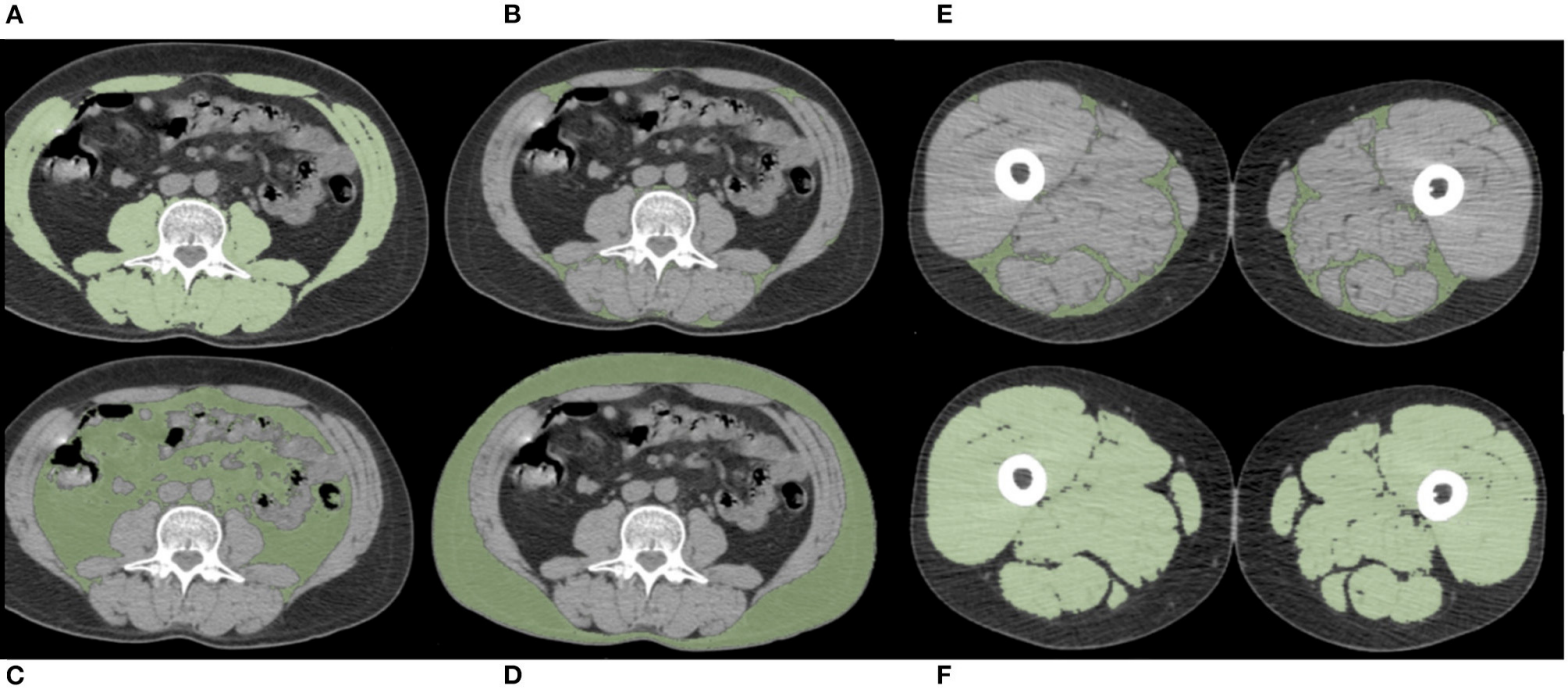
Examples of PET-CT body composition measurements. Muscle and adipose tissue segmentations were obtained after applying specific density thresholds. In these axial slices at the level of the third lumbar vertebra (L3) (A–D) and at the level of proximal thigh (PT) (E,F), the colored regions of interest represent L3- and PT-skeletal muscle area (SMA) (A,F), L3- and PT-intermuscular adipose tissue (IMAT) (B,E), visceral adipose tissue (VAT) (C), and subcutaneous adipose tissue (SAT) (D).
